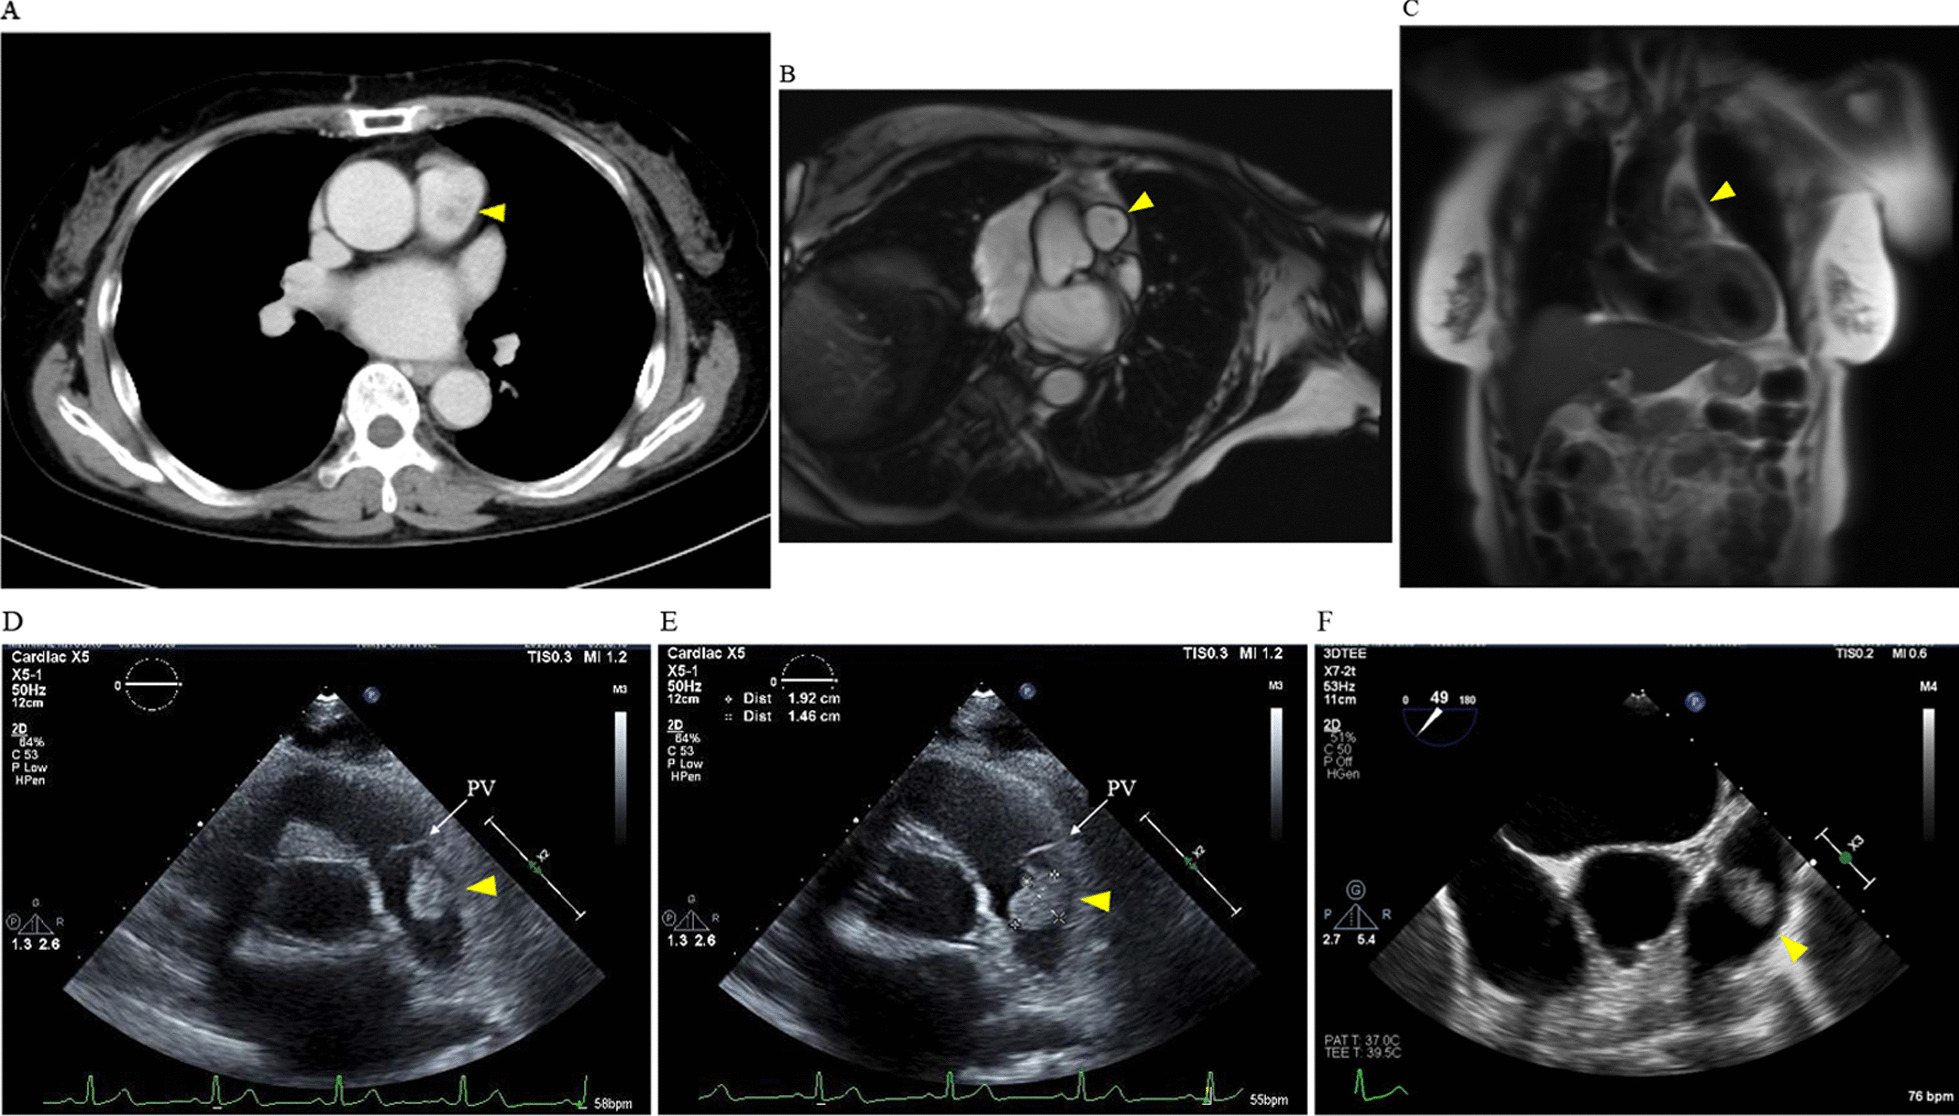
Preoperative findings. A Computed tomography. B, C Magnetic resonance imaging. D, E Transthoracic echocardiography. F Transesophageal echocardiography. (Each examination revealed a tumor shown as a yellow triangle)
